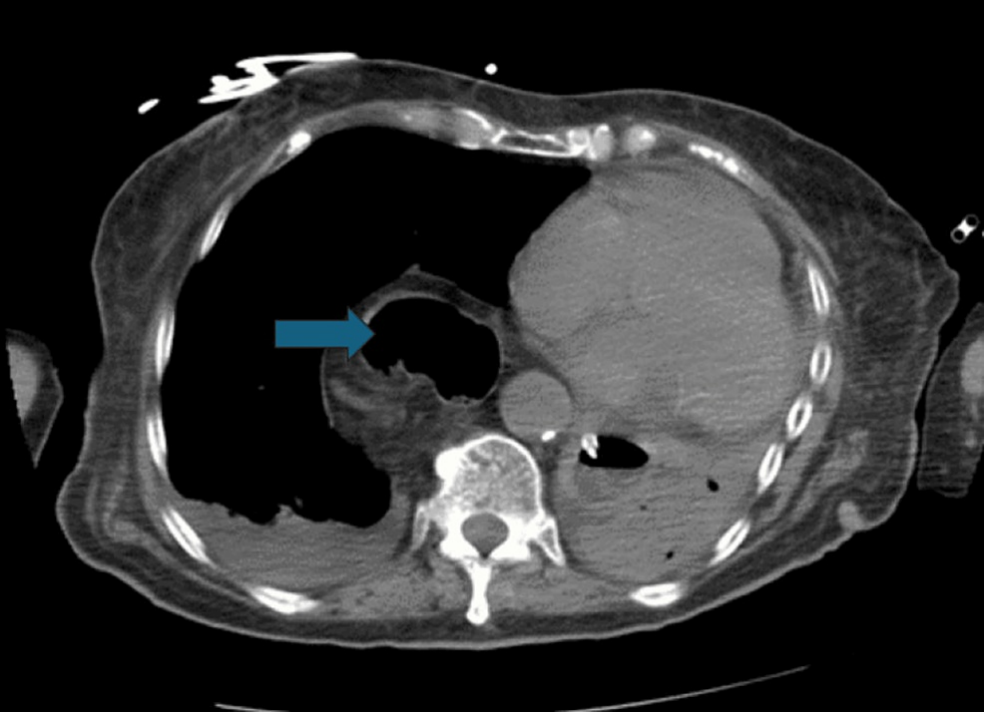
CT scan showing a dilated esophagus (blue arrow)
